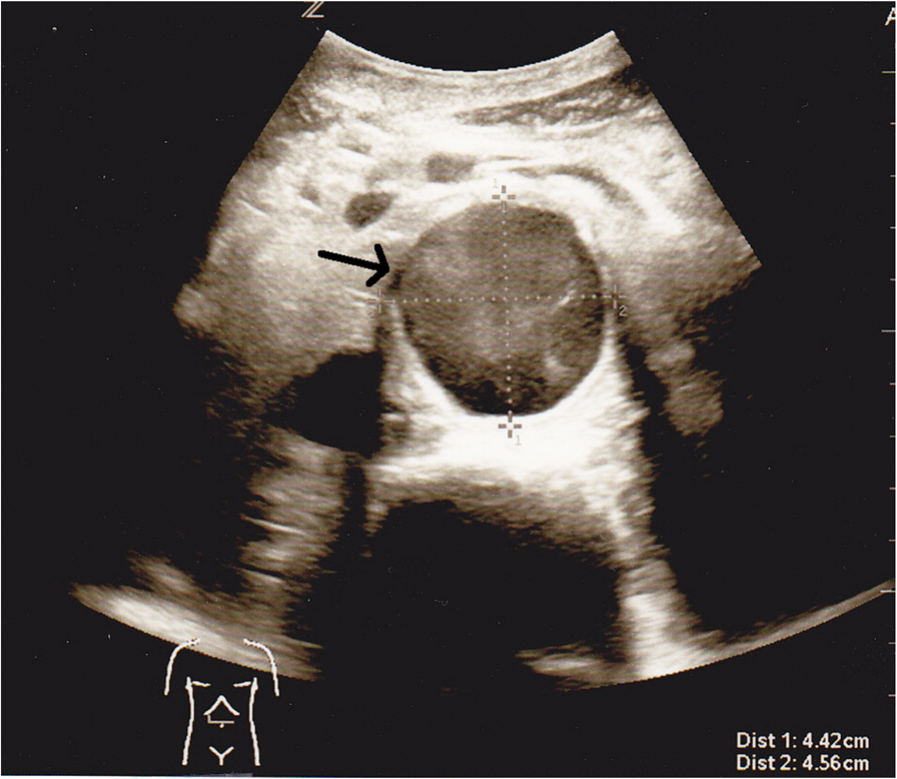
Ultrasound image diagnostic for abdominal aortic aneurysm.
